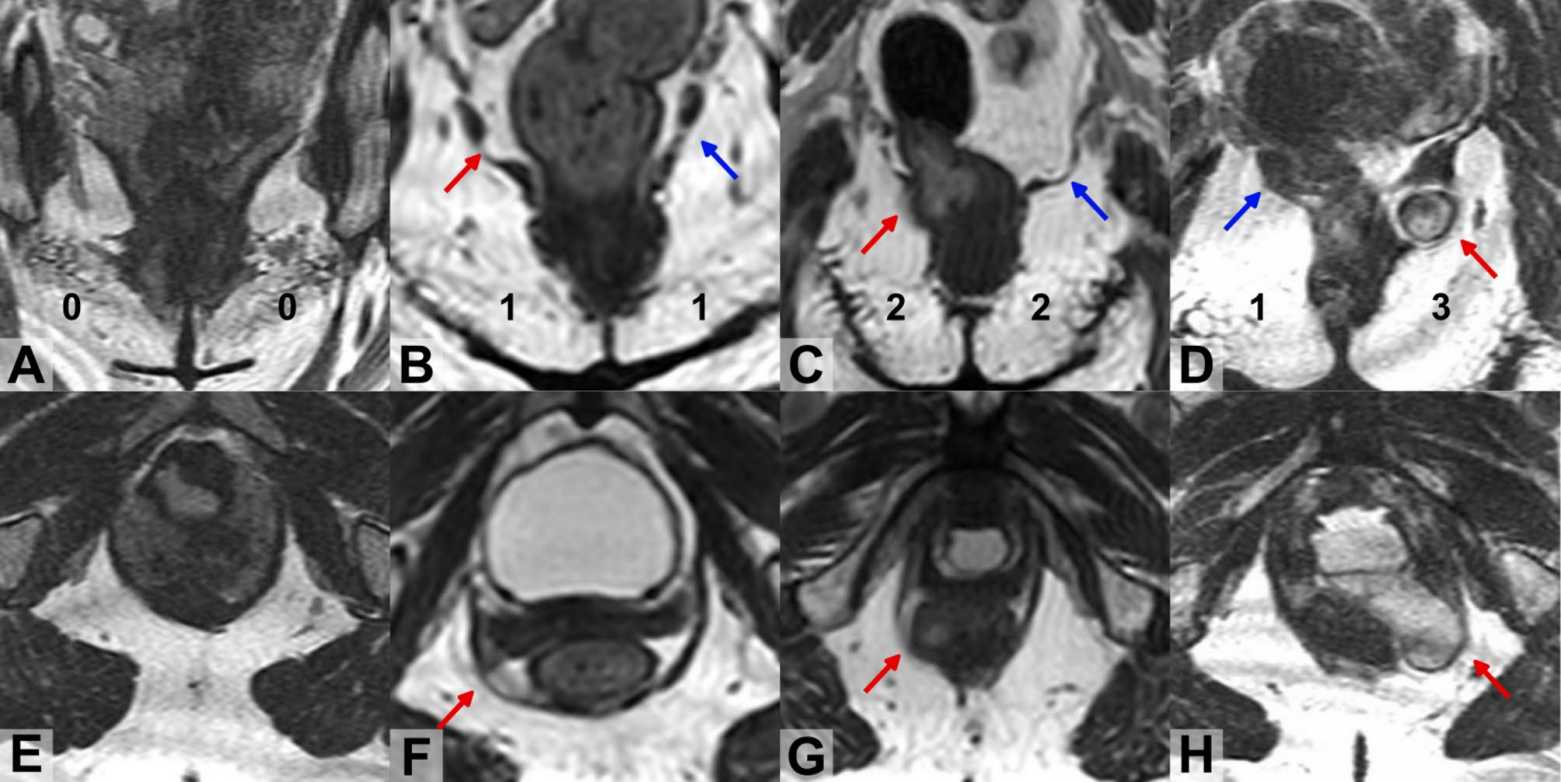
Examples of the appearance of the iliococcygeus muscle damage assessing scoring in coronal (A, B,C, D) and axial (E, F,G, H) MR images. A and E represents the normal shape of the muscle; B and F showed a woman with minor damage. C and G showed a woman with major damage. D and H showed a woman with major damage with hernia in the left side. Blue and red arrows point ICM damage in coronal view. In axial view, the damage pointed by the red arrow corresponds to the same damage pointed by the red arrow on coronal view.
